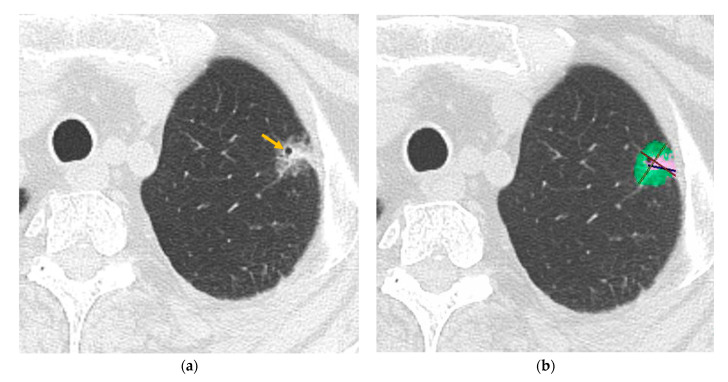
An 80-year-old woman with invasive adenocarcinoma (negative for STAS). (a) HRCT shows a part-solid nodule with an air bronchogram (arrow). The diameter of the tumor is 16.6 mm, and the diameter of the solid component is 8.0 mm. The rate of the solid component diameter is 48.2%. (b) The volume of the tumor and solid component are 1650.9 mm^3^ (pink and green area) and 325.0 mm^3^ (pink area), respectively. The rate of solid component volume is 19.7%. Note—STAS, spread through air spaces; HRCT, high-resolution CT.
